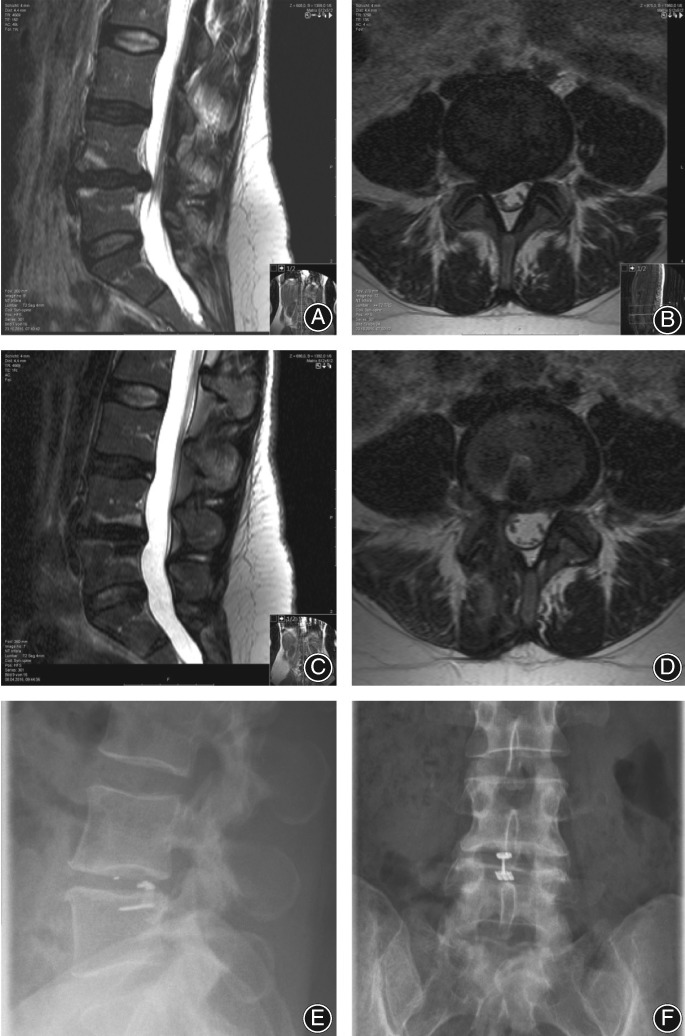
Lumbar disc hernia L_4/5_ right in a 40‐year old woman (A, B). The sequester (1.3 mL) was completely removed and the anular defect (4 mm height and 8 mm width) was closed by the anular closure device. (C, D) MRI 5 months postoperatively. Postoperative x‐rays show a correct position of the implant 2 mm ventral to the posterior wall of the vertebral body and anchor in L_5_ (E, F). The iridium marker of the occlusion component reveals a correct position of the device within the disc space (E).
